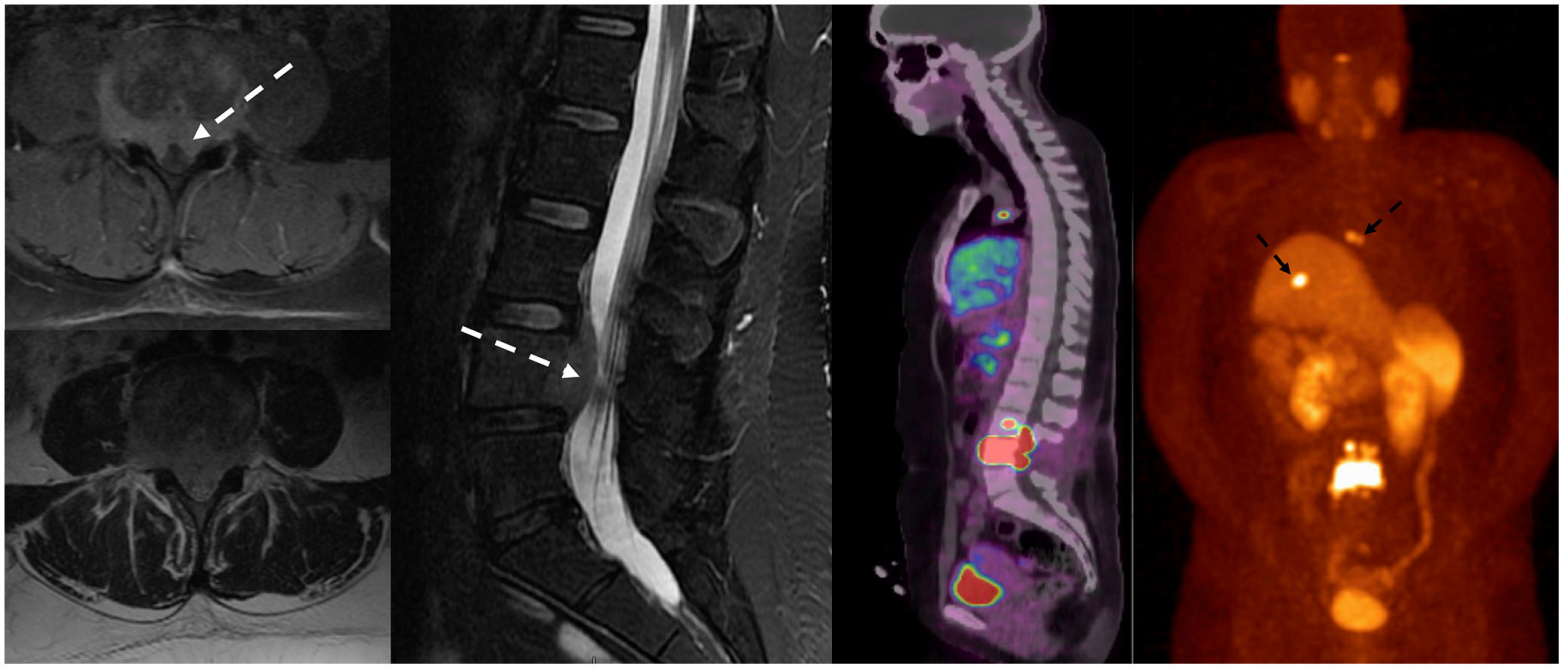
Patient with a history of lung carcinoid tumor status post-surgical resection presented with back pain. MRI of the lumbar spine demonstrated lumbar spinal metastasis with ventral epidural extension partially effacing the thecal sac at L4 (dotted white arrow). Ga-68 DOTATATE PET/CT showed avid DOTATATE uptake of the lumbar spinal metastasis and additional metastatic lesions in the right peritracheal lymph node and the liver (dotted black arrow).
